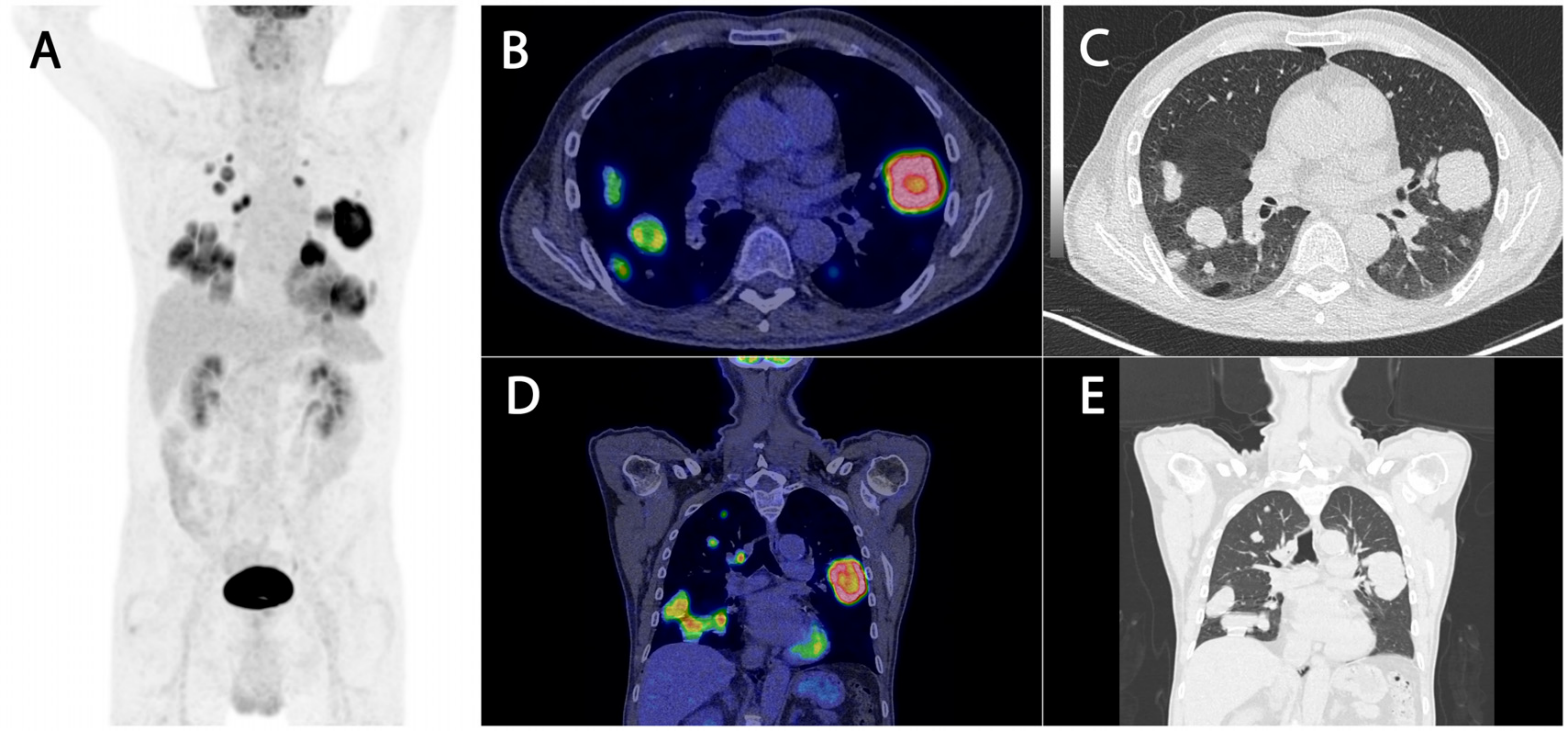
(A) Whole-body maximum intensity projection (MIP) PET image reveals multiple large pulmonary nodules bilaterally and mediastinal lymphadenopathy. The largest nodule, located in the right lower lobe, measures 7 cm. (B-E) Axial (B-C) and coronal (D-E) CT and corresponding positron emission tomography/computed tomography PET/CT images from 2023 demonstrate intense fluorodeoxyglucose (FDG) uptake in multiple pulmonary nodules and right hilar lymph nodes, consistent with metabolically active metastatic disease.
